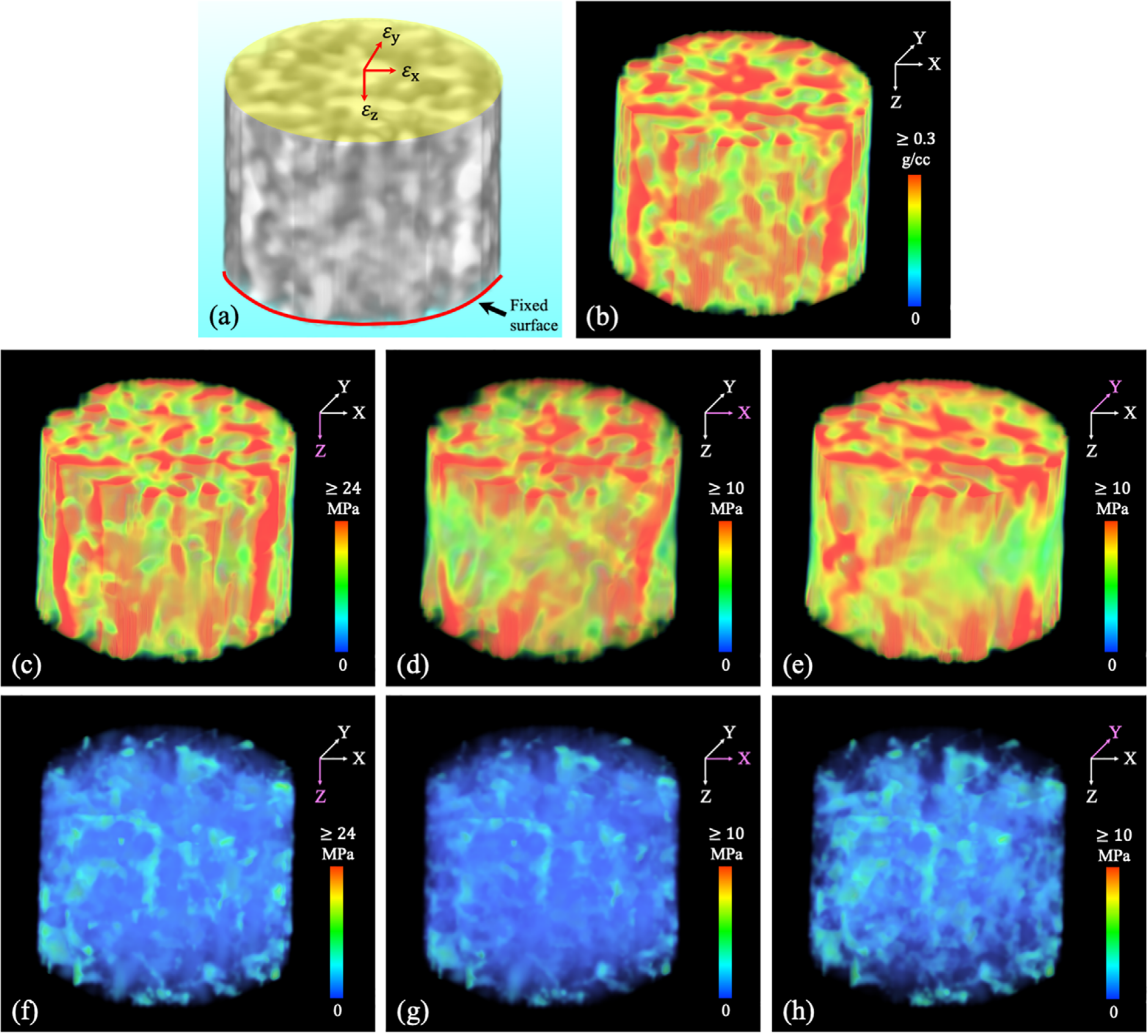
CT‐based FEA of Tb microstructure at different loading conditions. (*A*) Boundary and loading conditions for FEA on a cylindrical Tb core. (*B*–*E*) Color‐coded volume rendition of CHA density distribution (*B*), von Mises stress distribution under compressive (*C*), and x‐ and y‐ shear (*D*,*E*) loading conditions. (*F*–*H*) Stress leakages over marrow space under different loading conditions. Maximum stress value of 24 MPa is used for compressive stress distributions (*C*,*F*), whereas 10 MPa is used for shear stress distributions (*D*,*E*,*G*,*H*).
